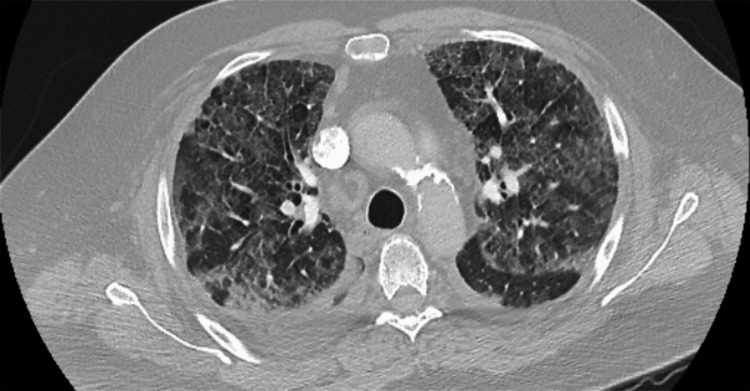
A CT of the chest showing diffuse interlobular septal thickening with superimposed patchy ground-glass opacities or “crazy-paving” pattern CT, computed tomography
